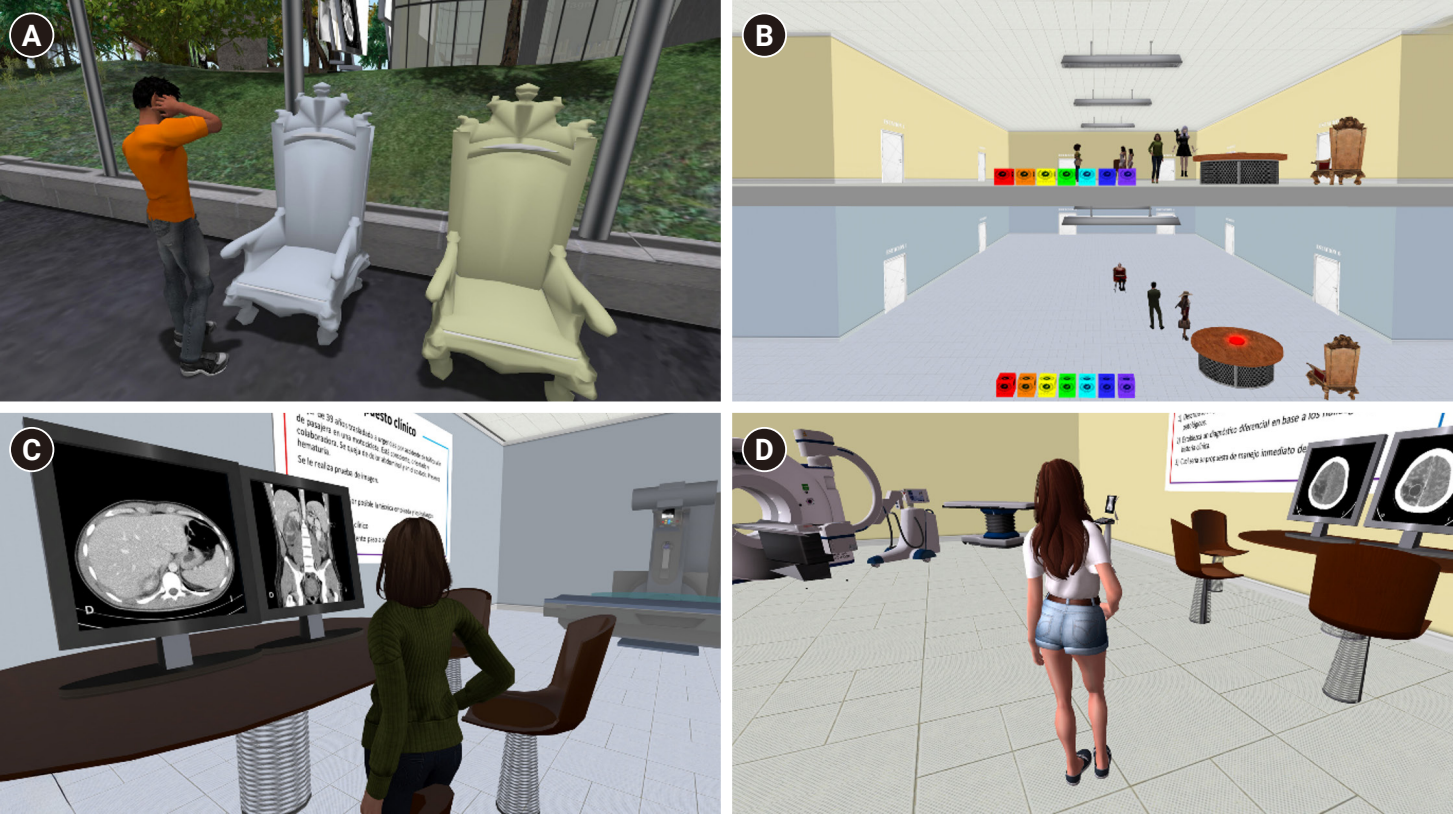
Various scenes from the virtual objective structured clinical examination (OSCE) experience in Second Life. (A) An avatar next to 2 chairs that teleport to the OSCE rooms. (B) View of the hallways of the Blue and Yellow OSCE rooms. (C) OSCE station in the Blue Room, showing a student viewing 2 monitors with abdominal computed tomography images. (D) OSCE station in the Yellow Room, showing a student reviewing neurology emergency case.
